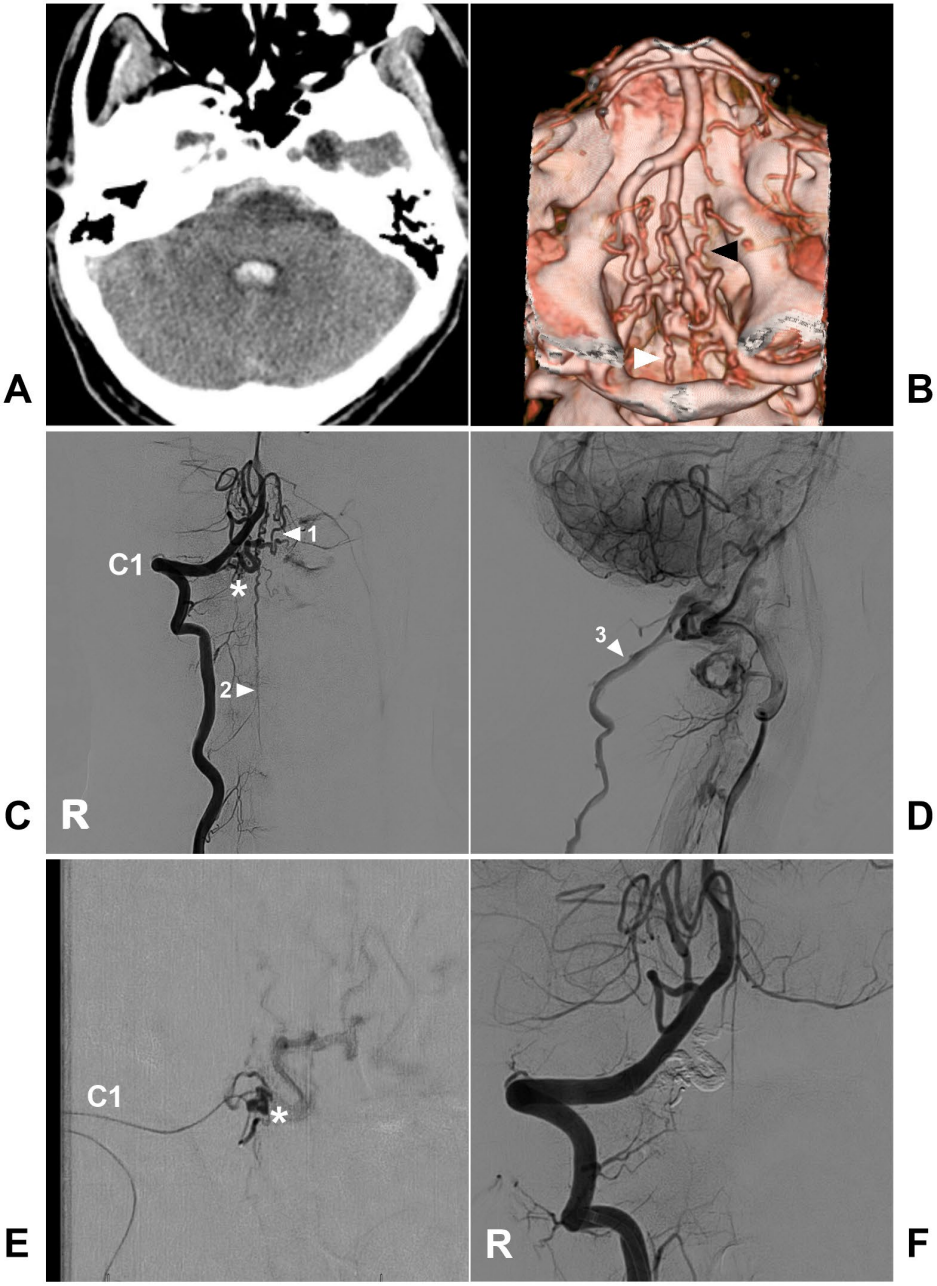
Complete EVT in a CCJ DAVF with a single feeding artery. (A) CT showing subarachnoid hemorrhage and fourth ventricle hemorrhage. (B) CTA showing multiple dilated abnormal veins (arrowheads). (C) Arterial phase DSA of the right VA showing a CCJ DAVF (asterisk) fed by the C1 radicular artery. The drainage went upward into the intracranial vein (arrowhead with number 1) and downward into the perimedullary vein (arrowhead with number 2). (D) Venous phase DSA of the lateral view showing drainage into the deep jugular vein (arrowhead with number 3). (E) Selective angiography of the microcatheter via the C1 radicular artery showing that the fistula was a DAVF (asterisk), and the microcatheter was obtained in the wedge position to perform Onyx casting. (F) Postoperative DSA showing that the DAVF was obliterated completely. C1, first cervical vertebrae; CCJ, craniocervical junction; CT, computed tomography; CTA, CT angiography; DAVF, dural arteriovenous fistula; DSA, digital subtracted angiography; EVT, endovascular treatment; R, right; VA, vertebral artery.
